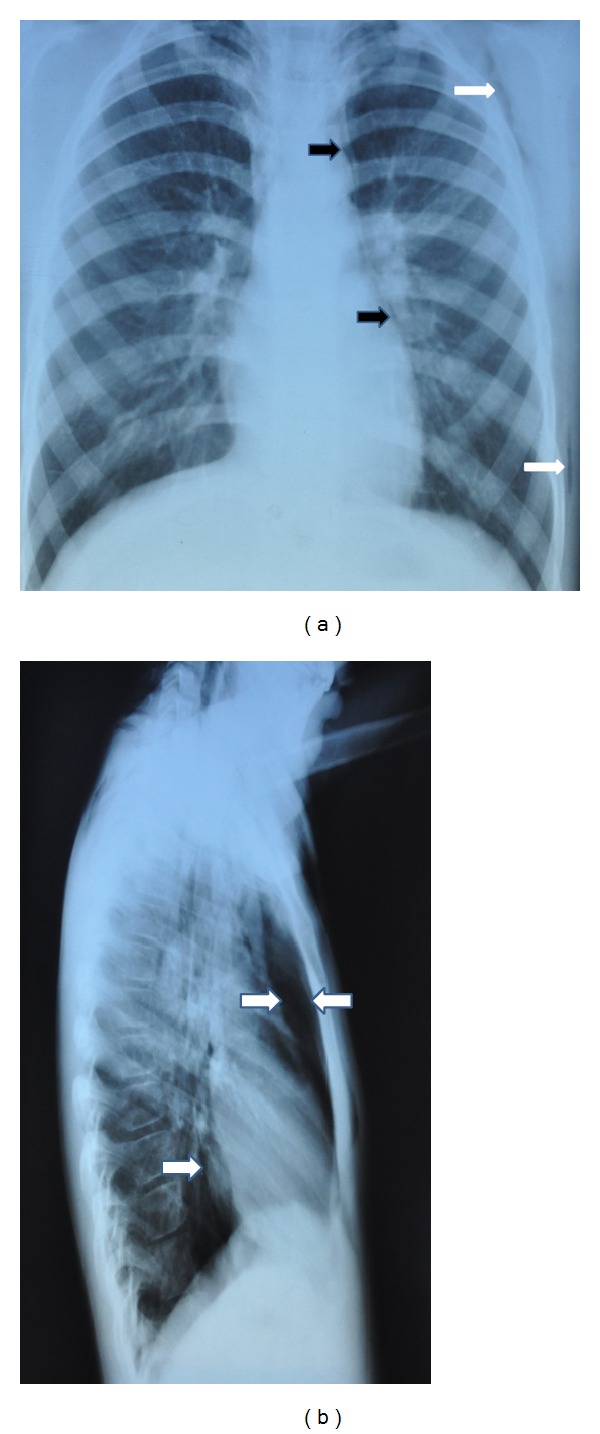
(a) Anteroposterior chest radiograph showing thin radiolucent line outlining aortic root and left heart border (black arrows) and subcutaneous emphysema (white arrows). (b) Lateral chest radiograph showing retrosternal emphysema (between arrows) and radiolucent line outlining posterior border of the heart.
